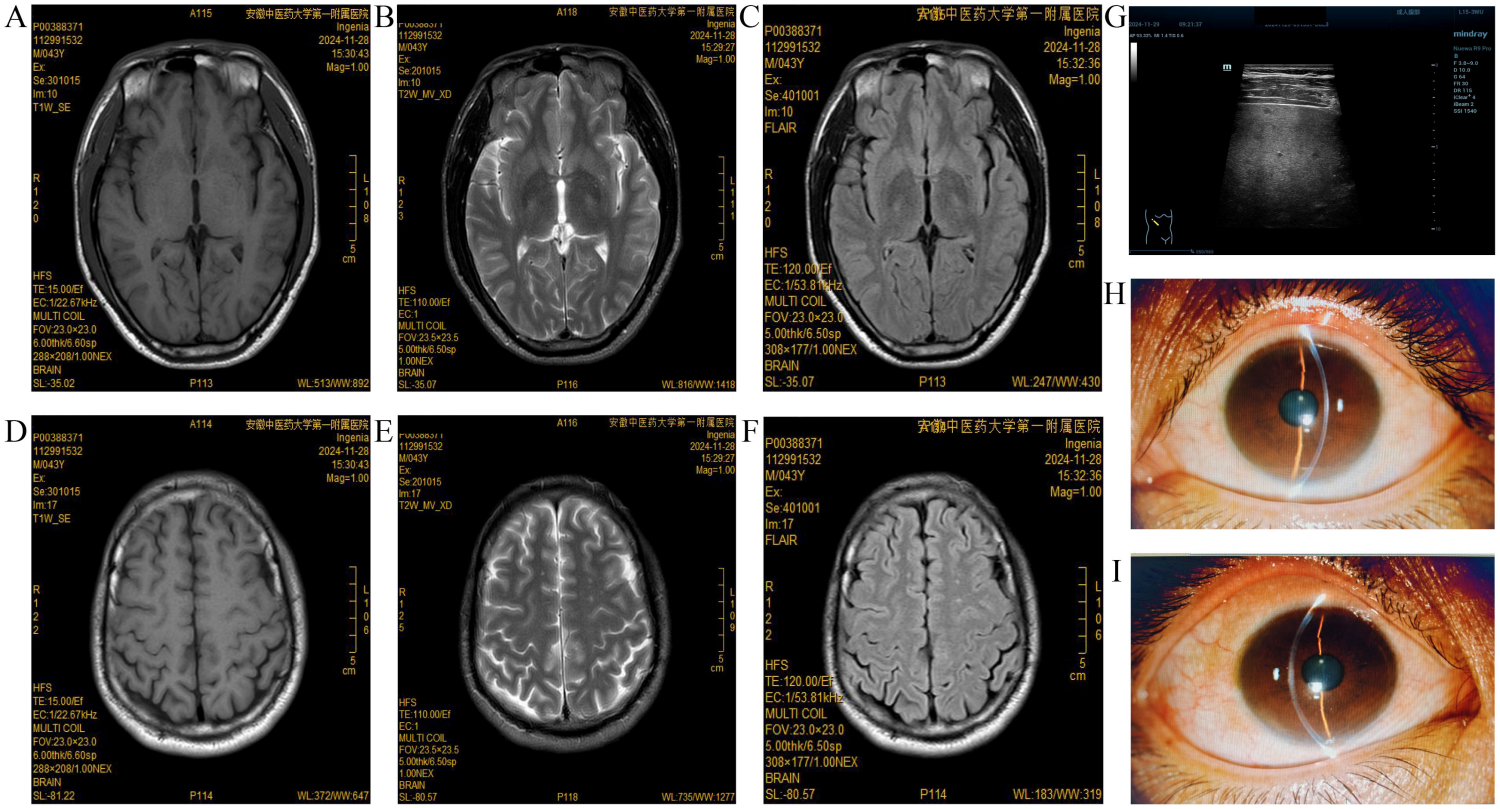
Brain MRI, liver ultrasonography and corneal K-F ring examination. (A–C) T1, T2, and Flair high signal in the left lentiform nucleus on the brain MRI plain scan; (D–F) Multiple speckled T1WI or slightly lower T2WI high signal shadows, with a high signal on FLAIR in the bilateral cerebral cortex on brain MRI plain scan; (G) Multiple hyperechoic nodules scattered in the liver; and (H, I) Few corneal K-F rings observed during slit-lamp examination.
